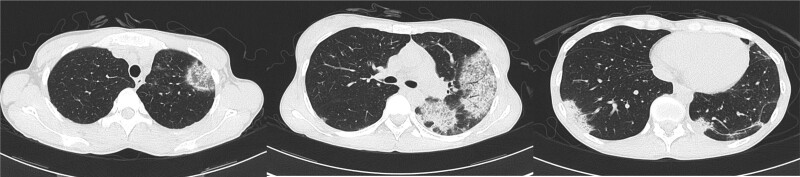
Chest computed tomography showing multiple bilateral peripheral patchy ground-glass opacities.
